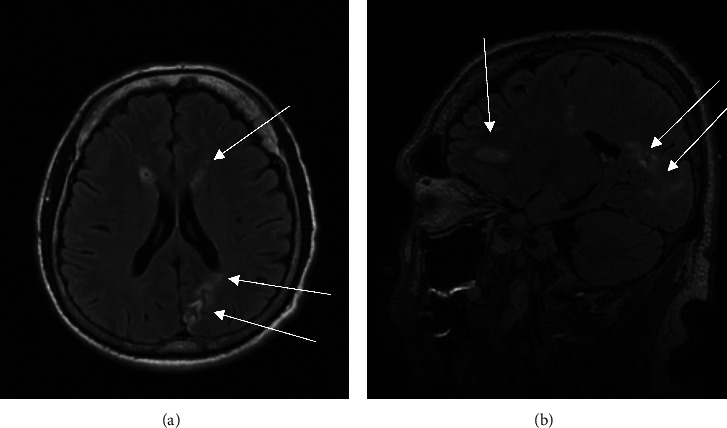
Repeat (a) axial and (b) sagittal views of MRI imaging after 12 weeks of antibiotic therapy demonstrated resolving left frontal, occipital, and parietal lobe abscesses.
